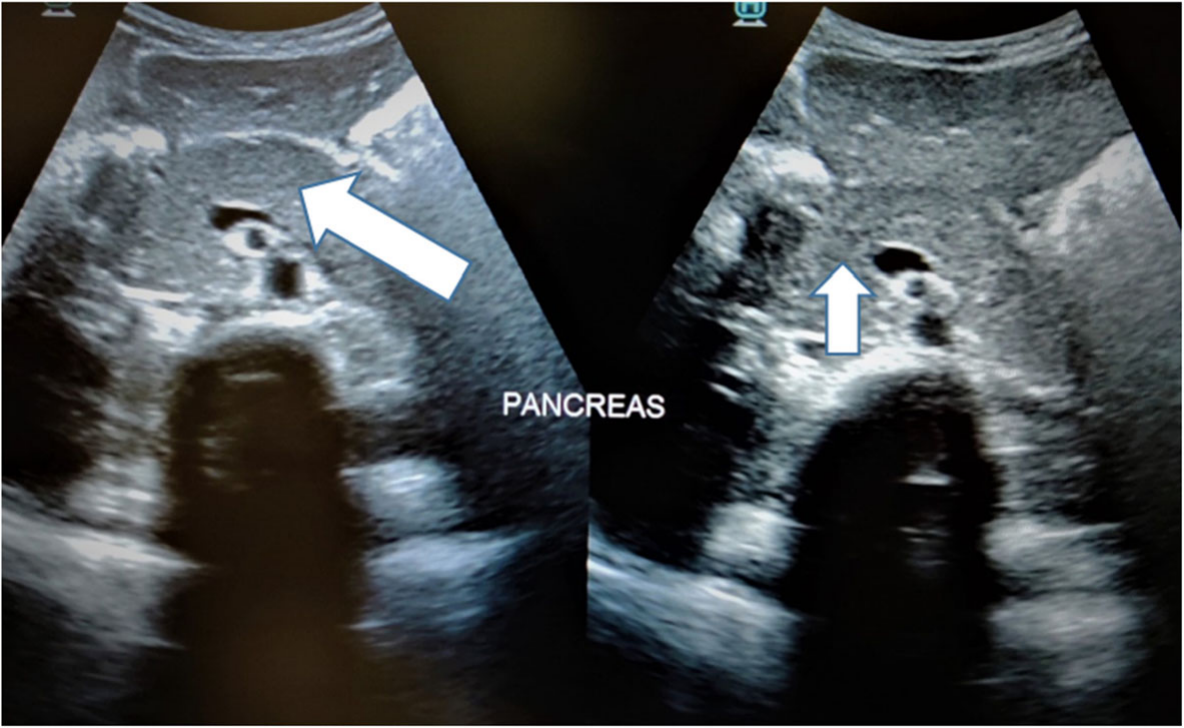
Grey scale ultrasound image showing features suggestive of acute pancreatitis: slightly bulky and enlarged visualized part of pancreas appearing uniformly hypoechoic. Main pancreatic duct appears normal. Minimal fat stranding was also noted
